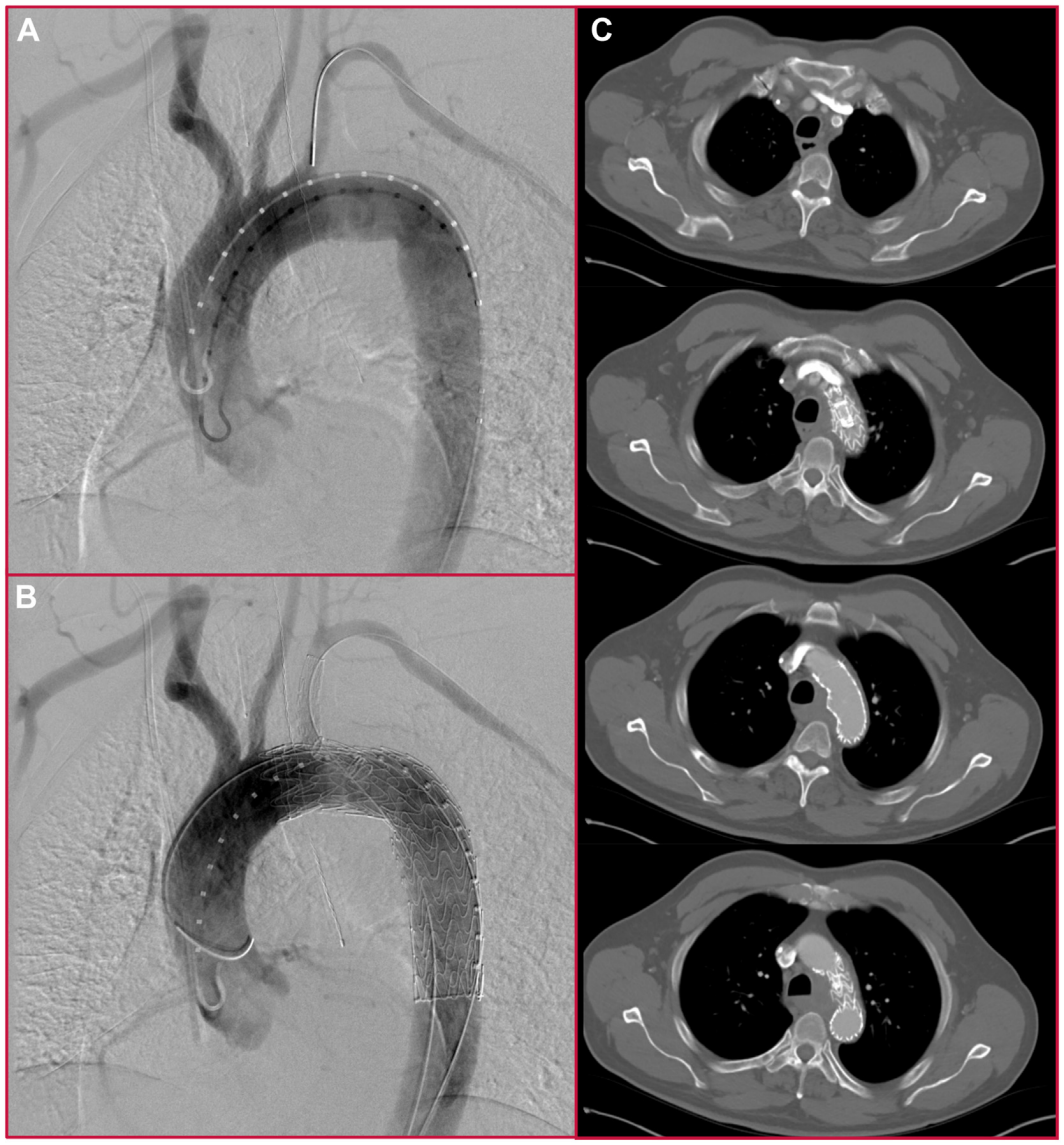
Intraoperative angiography. (A) Predeployment thoracic aortogram. (B) Completion thoracic aortogram. (C) Postoperative computed tomography (CT) angiogram revealing exclusion of posteromedial aortic pathology.
